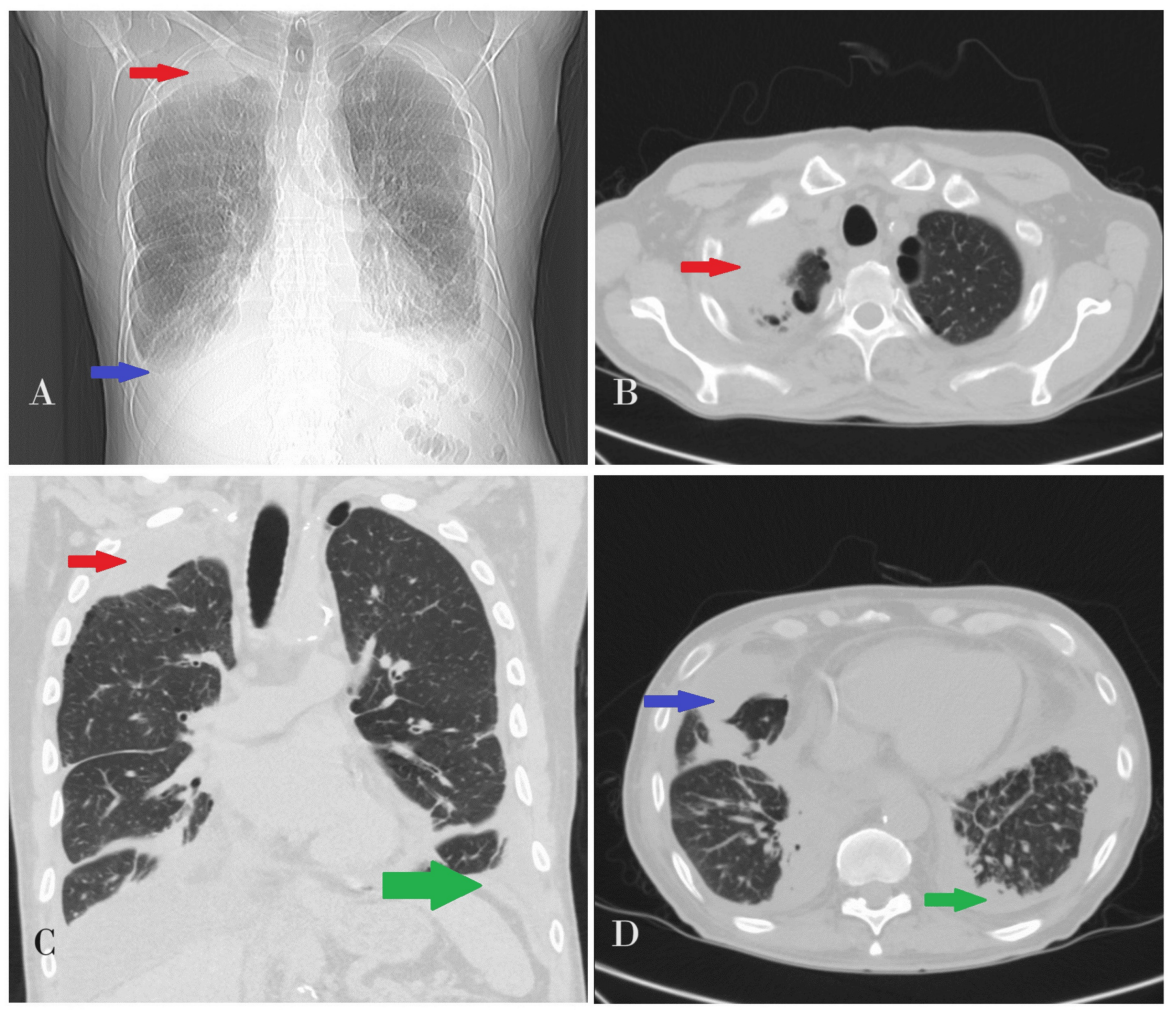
Chest computed tomography scan showing (A and C) pleural effusion in the right apex anteriorly with atelectasis pointed with a red arrow and consolidation in the right lower lobe and left lower pleural effusion with adjacent atelectasis/consolidation pointed with blue and green arrows, respectively; (B) axial view, right-sided partially loculated pleural effusion tracking into the right apex anteriorly pointed with a red arrow; and (D) axial view, atelectasis and consolidation in the right lower lobe and a small left pleural effusion with adjacent atelectasis/consolidation pointed with green and blue arrows, respectively
